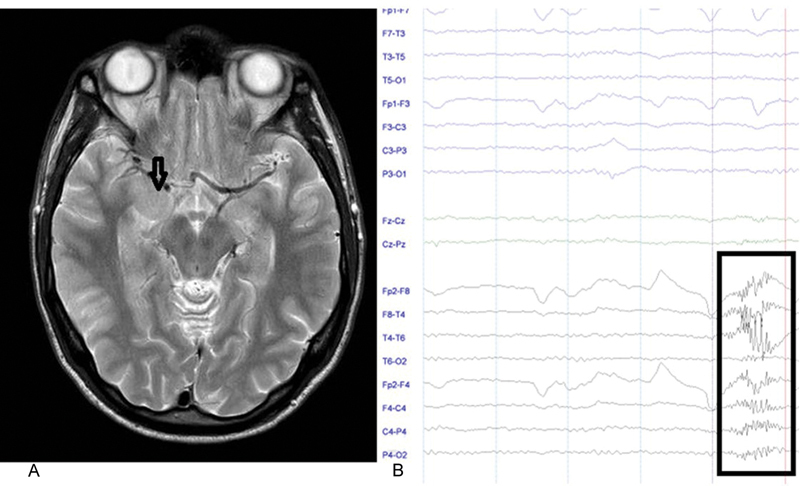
A: Axial T2-weighted brain MRI demonstrating right amygdalar enlargement (black arrow). B: Scalp EEG of the same patient showing bursts of short-interval polyspikes with a focus in regions F8 to T4 and T4 to T6 (inset). (Author's property).
